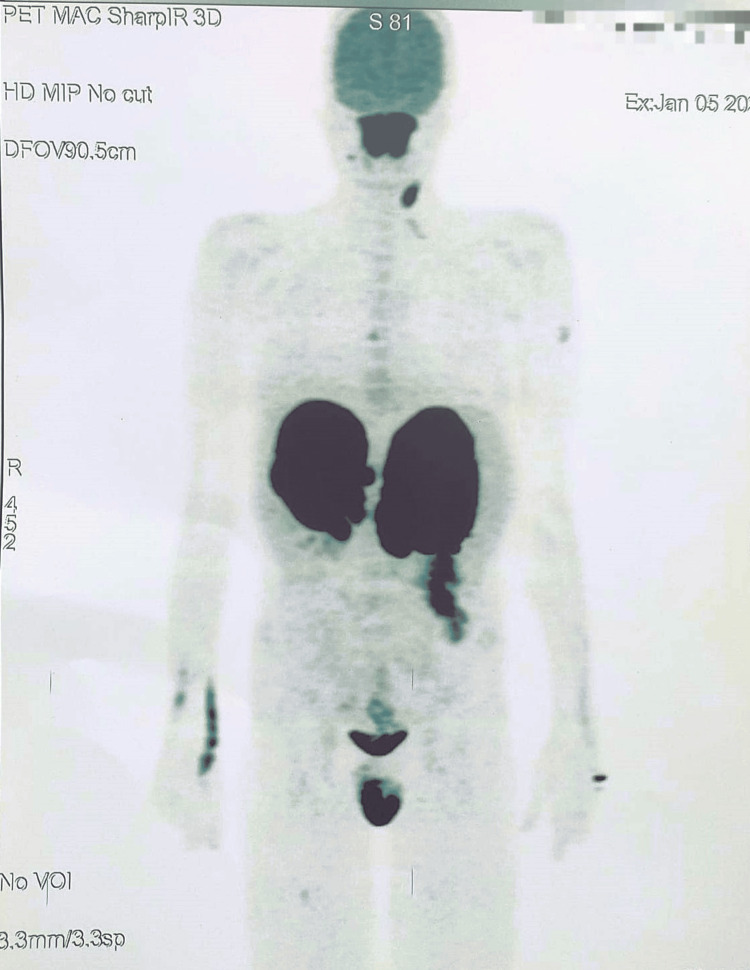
PET scanner Hypermetabolic thickening of nasal, maxillary, and ethmoidal sinuses, associated with active foci of bilateral cervical lymph nodes. Hypermetabolic tumor masses of the adrenal glands with necrotic centers: right SUV = 13.2 of 100*117*156 mm; left SUV = 14.7 of 127*95*172 mm. Very intense diffuse pathological testicular hypermetabolism (SUV = 11.7). Diffuse pathological hypermetabolism of the upper left colon (SUV = 8). Hepatic SUV: 0.9. SUV: standardized uptake value.
